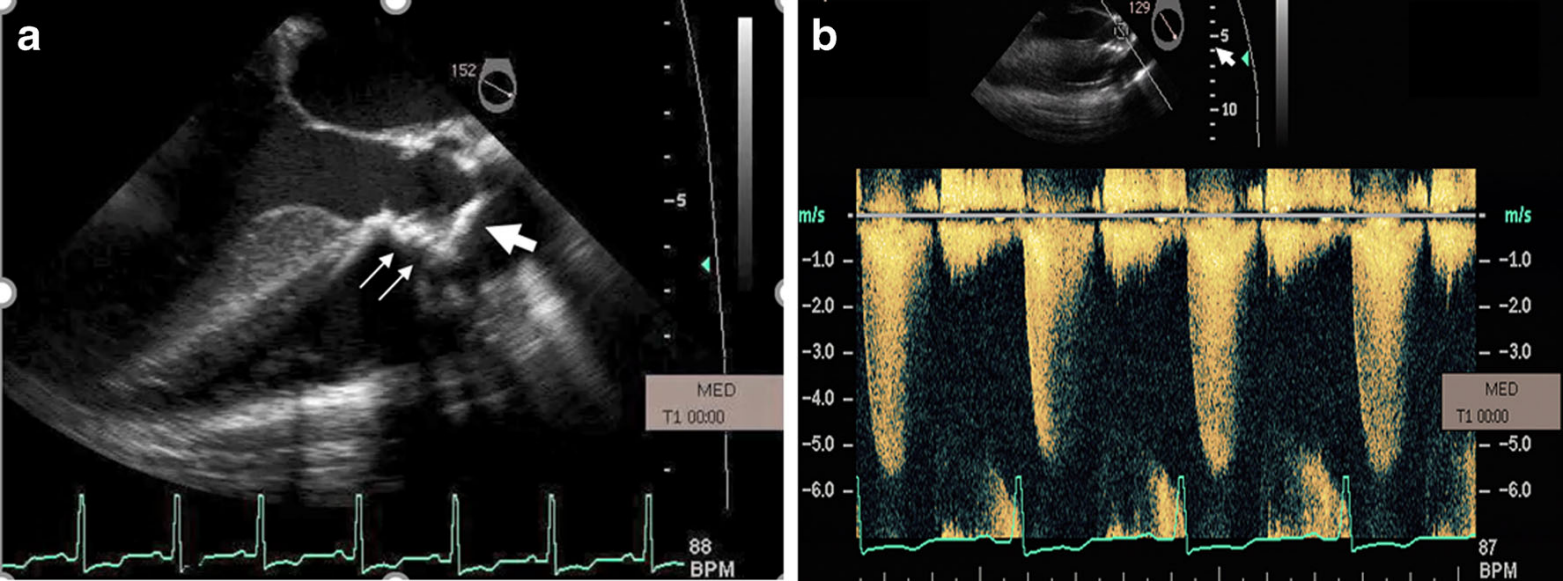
Thirty-two-year-old female with prior successful C-section 4 years ago developed hypotension postelective C-section at 37-week gestation. She had undergone bioprosthetic aortic valve replacement with a Carpentier-Edwards pericardial valve for severe aortic stenosis in a bicuspid aortic valve and aortic root enlargement with a pericardial patch due to a small aortic root 5 years ago. Due to dyspnea and an increase in mean prosthetic aortic valve gradient from 19 mm Hg pre-pregnancy to 51 mm Hg at 21-week gestation, an elective C-section was performed at 37 weeks. After extubation post-C-section, she developed pulmonary edema with a pulmonary capillary wedge pressure of 28 mm Hg and required pressor support. Transesophageal echocardiographic images showing calcified bioprosthetic aortic valve leaflets with restricted motion (*thick white arrow*) and prior pericardial patch (*thin white arrows*) in a and severely increased aortic valve gradient at 121 mm Hg in b
